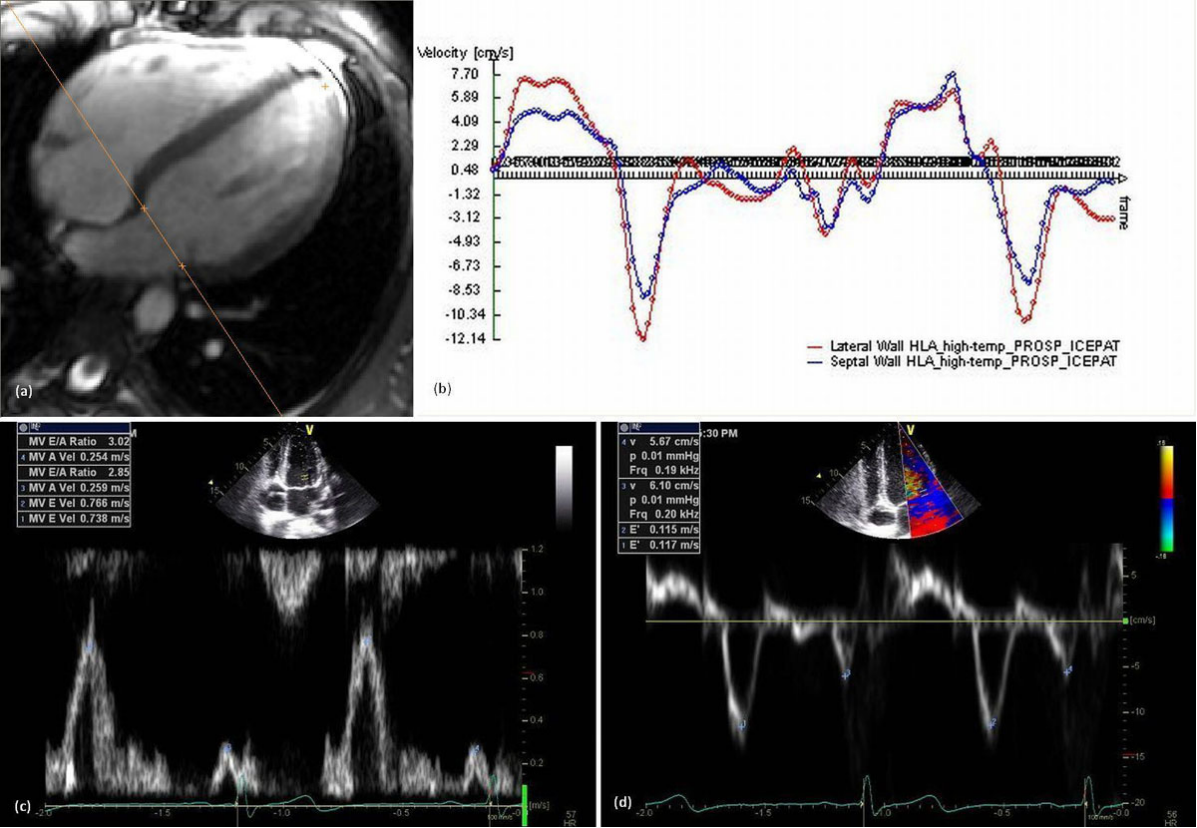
Mitral valve annulus velocity tracking in prospectively triggered MR (a), (b), mitral inflow velocity (c), and tissue velocities (d) from Doppler echocardiography. Mean lateral e’ velocities are 11.6 cm/s (echo) and 11.9 cm/s (CMR), and mean lateral a’ velocities are 5.8 cm/s (echo) and 4.9 cm/s (CMR).
